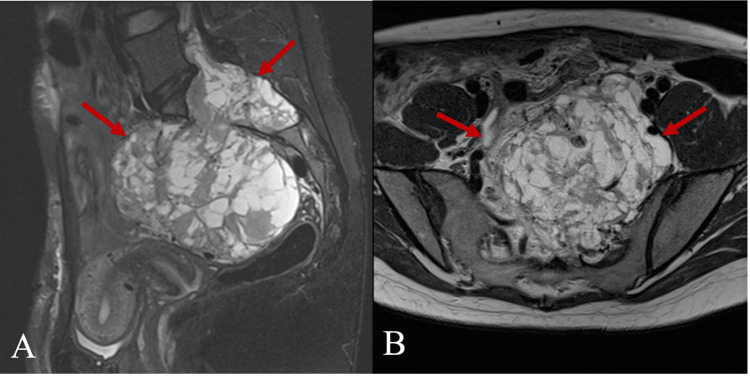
T2-weighted sagittal fat-saturated (A) and axial (B) MRI images show a huge sacral and presacral, well-defined, heterogeneous, and intermediate-to-high signal intensity tumor (arrows). Dislocation of adjacent structures (rectum, uterus, and vessels) is also visible. MRI, magnetic resonance imaging
